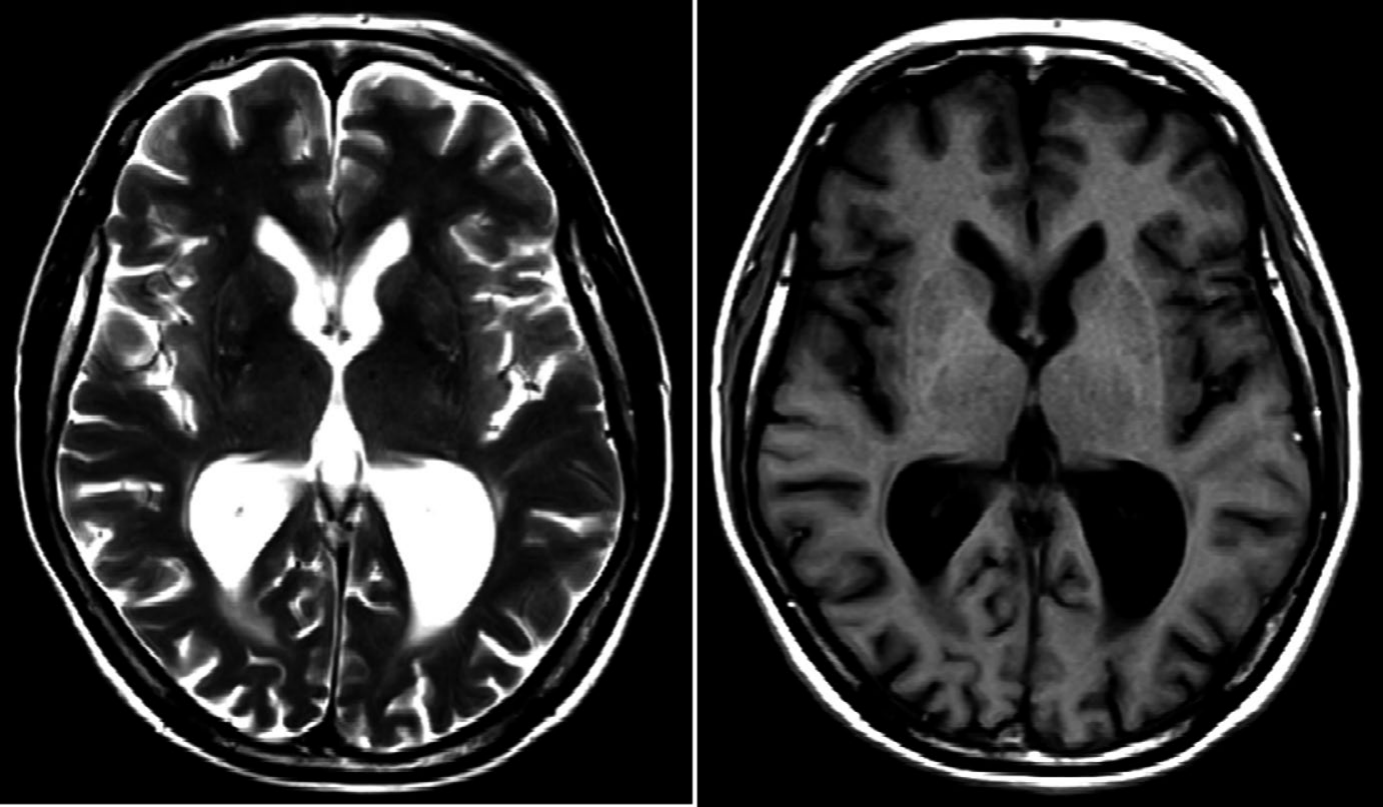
T1‐ and T2‐weighted magnetic resonance imaging exhibiting cerebral atrophy with putaminal accentuation and ventriculomegaly
